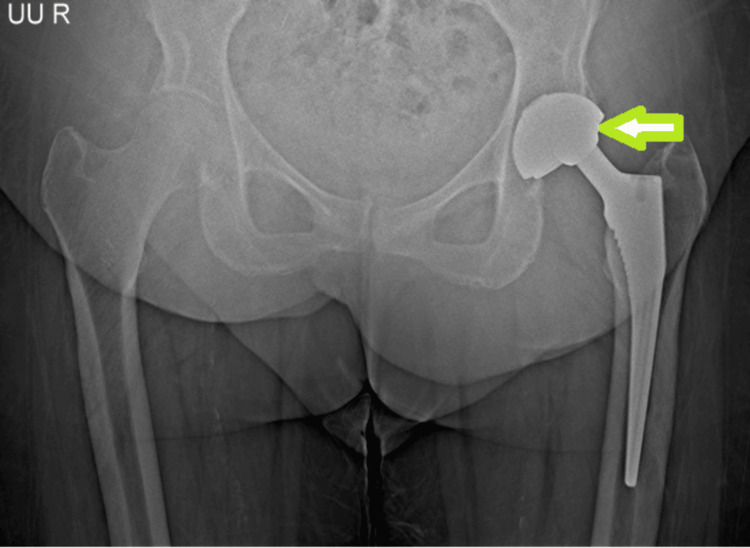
Radiographic imaging of the left hip postoperatively (two years after total hip arthroplasty). Anteroposterior (AP) view showing the position of the femoral component and acetabular shell. The green arrow demonstrates eccentric positioning of the femoral head within the acetabular component.
